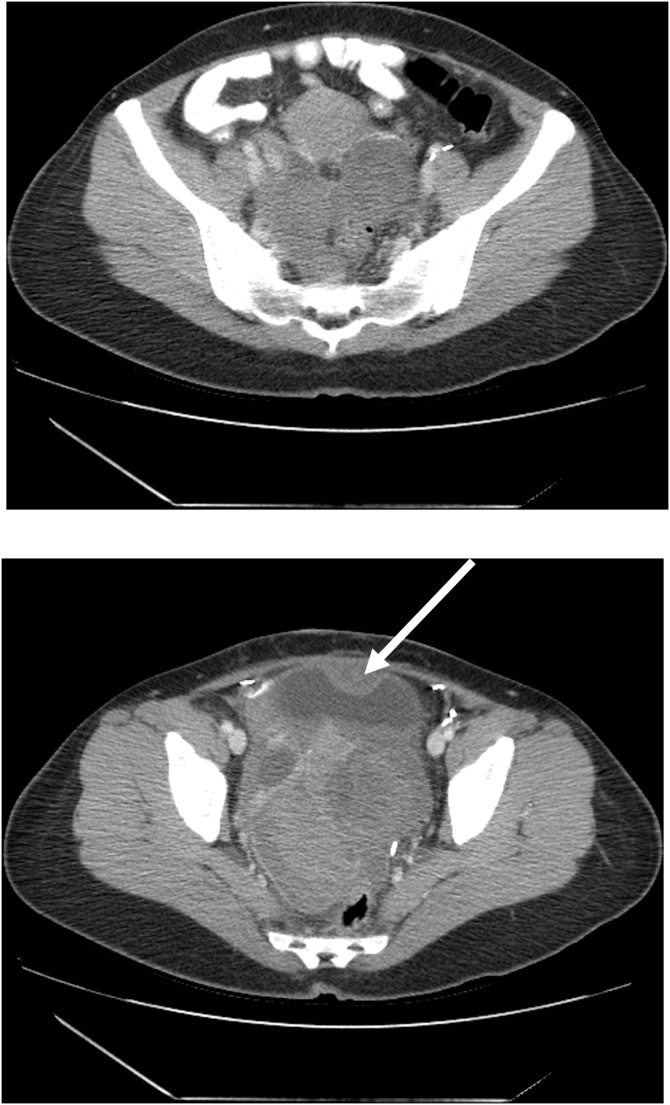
(top) CT shows a multilobulated mass greater on the left than the right. It is immediately adjacent to pelvic peritoneum on the right and covers the rectosigmoid junction on the left. It pushes the uterus superiorly. (bottom) CT shows a large mass that occupies the entire true pelvis. The rectum is markedly compressed. An arrow marks a separate sarcoma nodule at the dome of the bladder.
